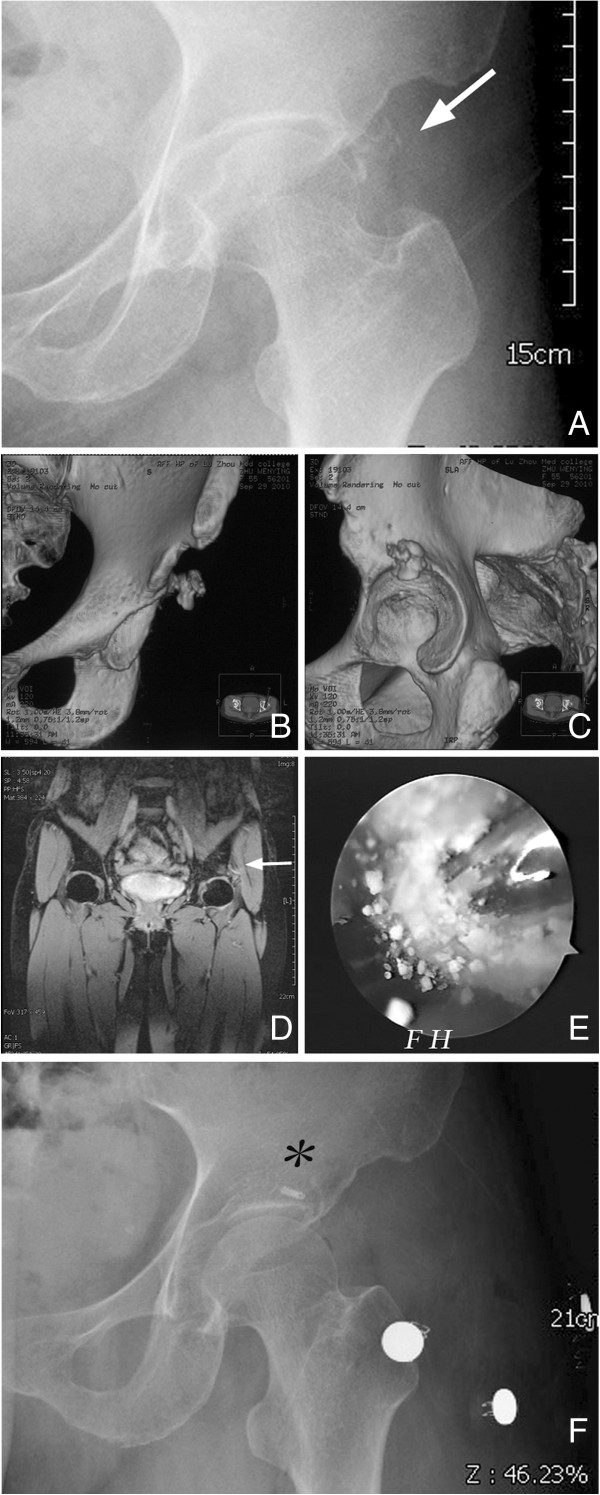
Case 3 radiographic data. (A) Anteroposterior (AP) projection of the left hip showing an ill-defined calcific spot (arrow) close to the acetabular roof. (B) Coronal and (C) sagittal three-dimensional reconstruction computer tomography images verified the large and irregular calcium mass. (D) The coronal enhanced fat-suppression phase arthro-MR slice showed miscellaneous signal changes of the origin of rectus femoris combined with labral tear (arrow). The tendon fibers had been displaced by the calcium mass and there was a large bone erosion of the acetabulum with peripheral soft-tissue edema. (E) Typical snowstorm-pattern after incision of the superficial capsular over the calcification (FH, femoral head). (F) Postoperative AP view demonstrating complete removal of calcific material; ^*^non-absorbable suture anchor for the labral repair.
